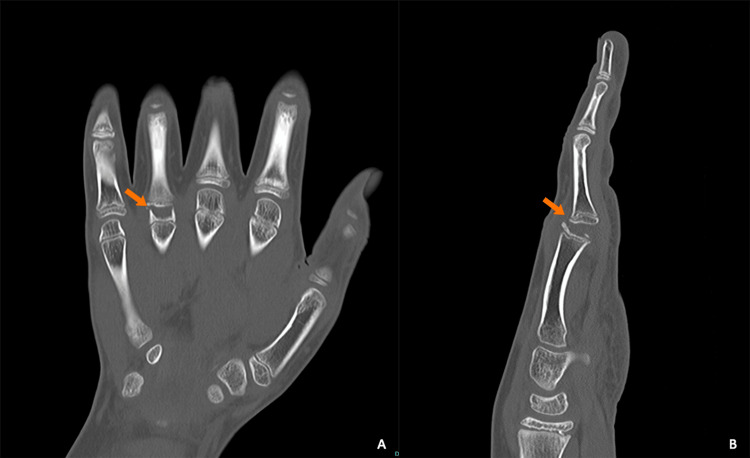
CT images of the left hand focusing on the fourth MCP joint in the bone kernel (a) Coronal CT of the left hand (bone kernel) displaying flattening and irregularity of the left metacarpal head (orange arrow). (b) Sagittal CT (bone kernel) depicting associated volar subluxation of the proximal phalanx (orange arrow) CT: computed tomography, MCP: metacarpophalangeal
